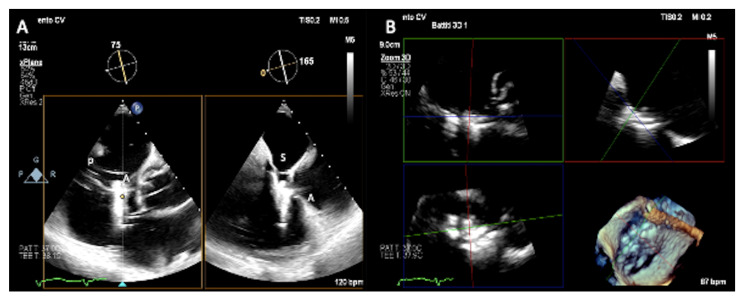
Intra-procedural monitoring by transesophageal echocardiography. (A) Right ventricular inflow-outflow view with biplane imaging to monitor septal (S) and anterior (A) leaflets grasping and (B) live 3D multiplanar reconstruction. A = anterior leaflet, S = septal leaflet, P = posterior leaflet.
